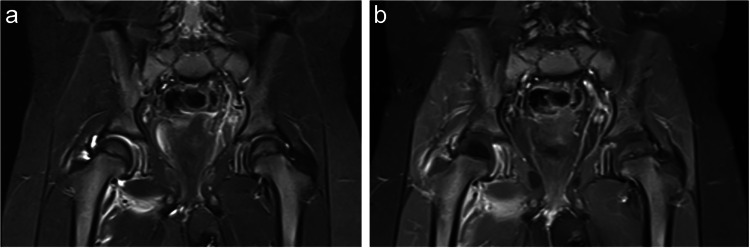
A 3-year-old girl with septic hip arthritis. The patient initially presented with 3 days of limping followed by a refusal to bear weight. She had a fever of 39.6 °C, a normal white blood cell (WBC) count, an erythrocyte sedimentation rate (ESR) of 80 mm/h, and a C-reactive protein (CRP) level of 4.3 mg/dL. Right hip aspiration revealed a WBC count of 175,000 cells/mL, with a positive culture for *Staphylococcus aureus*. A coronal MRI STIR shows moderate right hip effusion and edema in the adductor minimus muscle. B coronal MRI SET1 with fat suppression post-contrast shows decreased enhancement of the right femoral head
